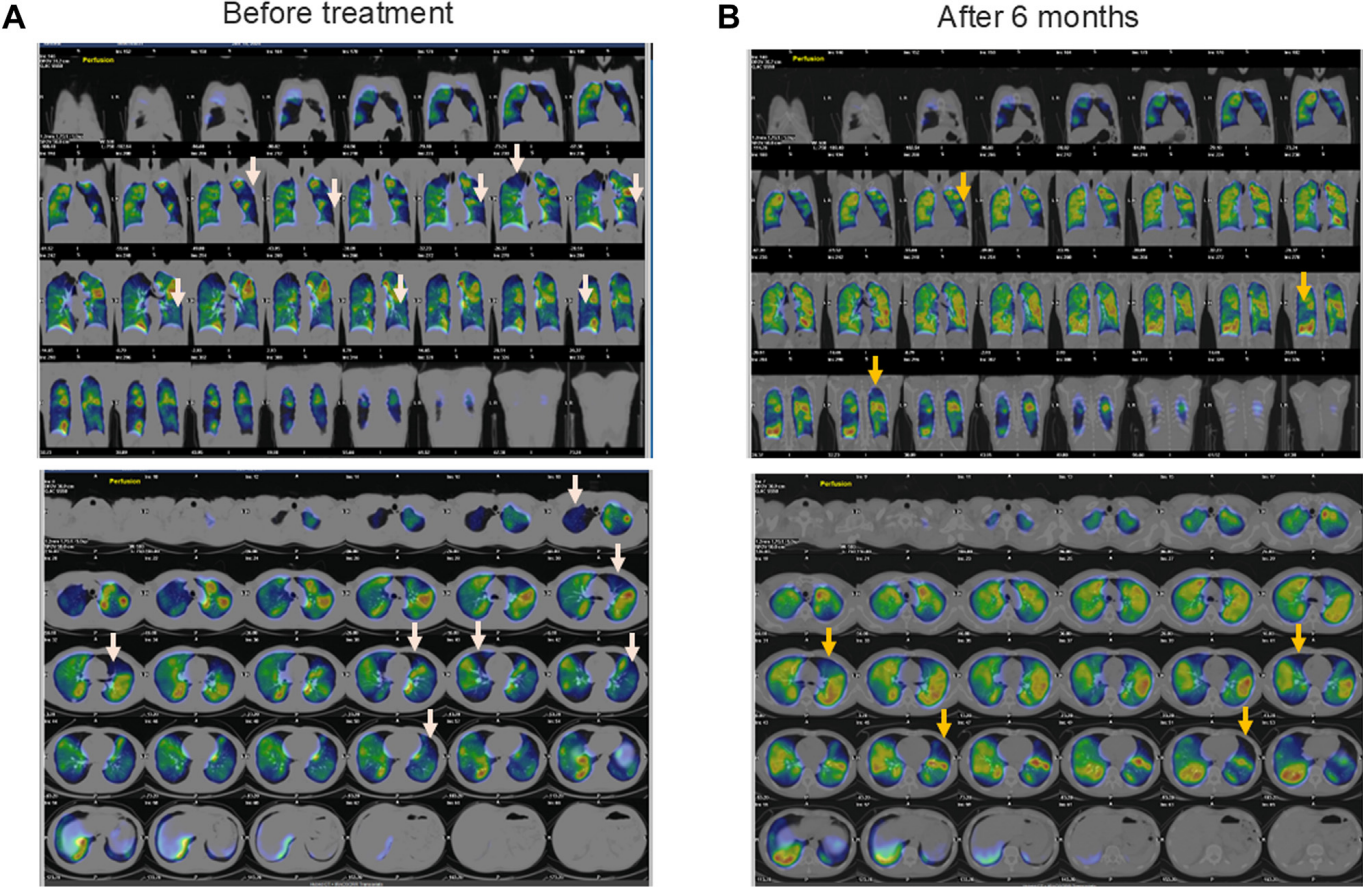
Pulmonary ventilation blood flow scintigraphy (A) before treatment and (B) after 6 months. Before treatment, the right lung showed defects in the apex, the right middle lobe, and the S6 and S10 regions of the right lower lobe; the left lung showed defects in the left upper segment and S8 region of the left lower lobe. After 6 months, significant improvement had occurred in both the pulmonary apex and the right S10 area. However, defects were still present in the right S2 and S6 areas, the right middle lobe, the left S1+2 and S9 areas, and the tongue area, although the severity of these defects had decreased. The light gray and yellow arrows indicate the defect area on pulmonary ventilation blood flow scintigraphy.
